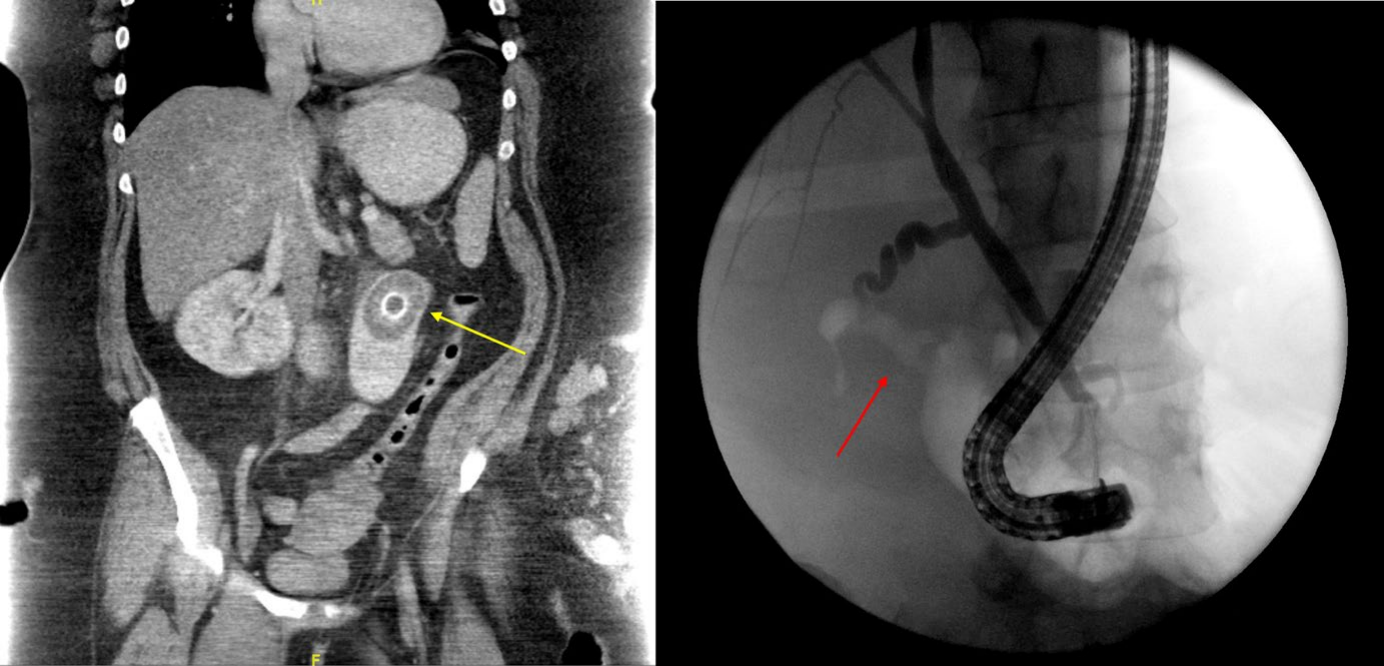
Left: Abdominal CT scan showing impacted gallstone in bowel lumen (yellow arrow). Right: Fluoroscopic image during ERCP showing cholecystoduodenal fistula (red arrow)
